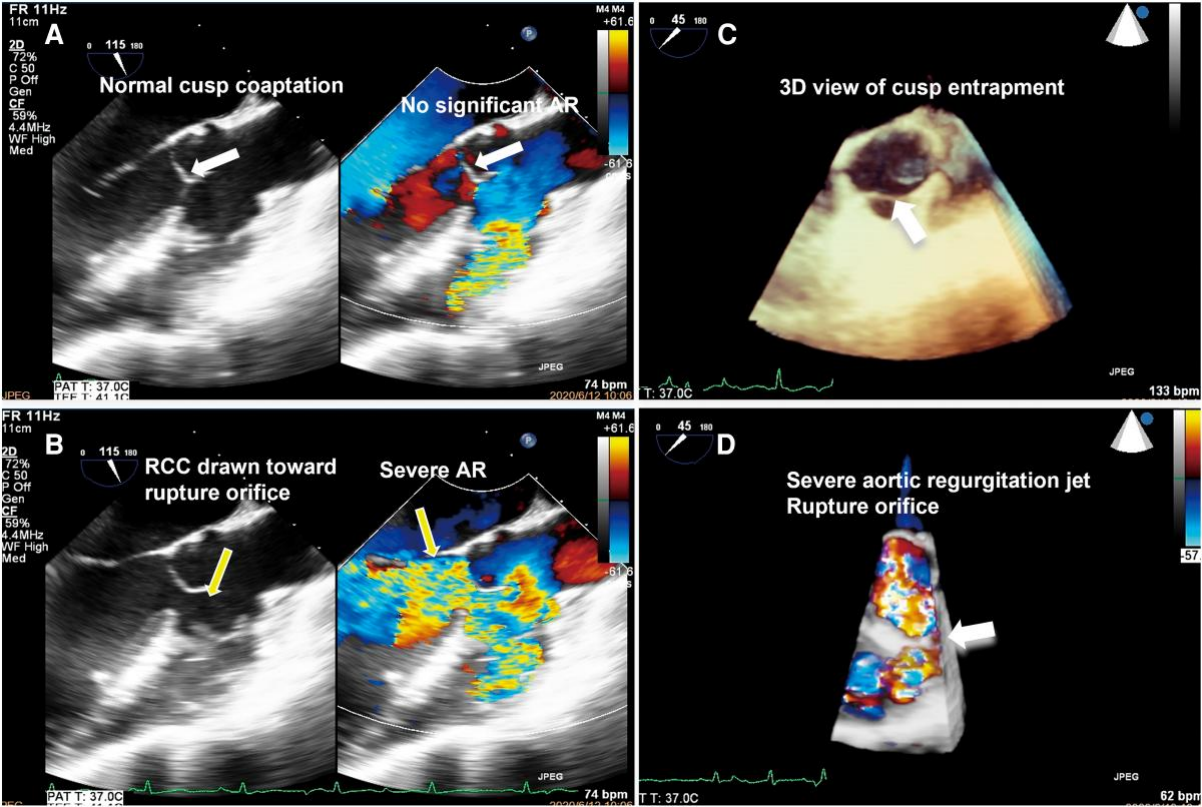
Three-dimensional transoesophageal echocardiography (3D-TEE) demonstrating intermittent severe aortic regurgitation caused by dynamic right coronary cusp entrapment at the rupture orifice. (*A*) Normal aortic valve closure without significant regurgitation (white arrow). (*B*) Suction-induced diastolic opening of the right coronary cusp with severe aortic regurgitation (yellow arrow). (*C* and *D*) Three-dimensional rendered views show intermittent entrapment of the right coronary cusp by the rupture defect, resulting in severe aortic regurgitation.
